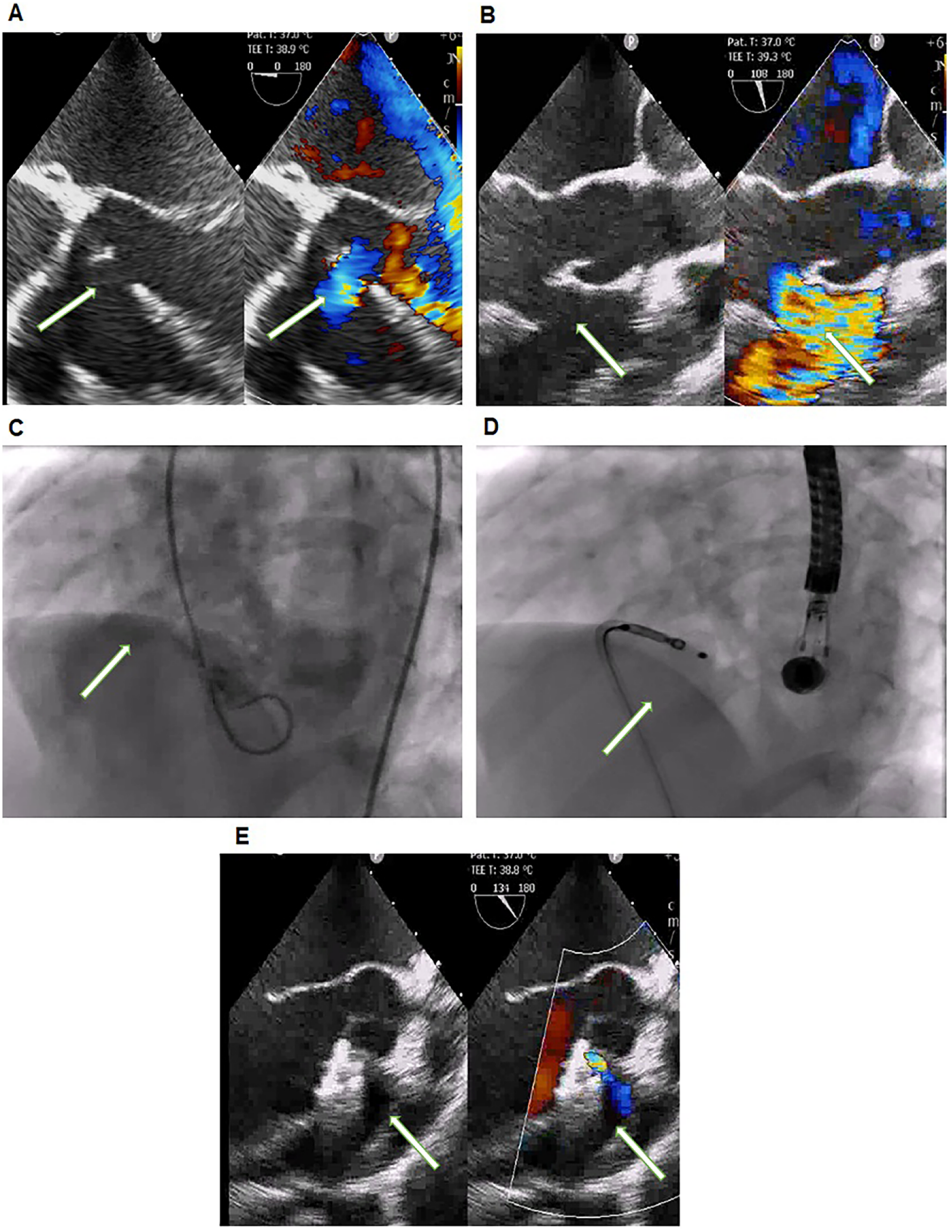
(A) TTE 0 degrees view with Doppler color and (B) TEE 110 degrees with Doppler color showing the perimembranous VSD (arrow). (C) Left Ventricular Angiography: the arrow shows the VSD. (D) The antegrade approach shows the device occluding the defect with the delivery cable attached to the RV disc. Post-device closure in (E) TEE 135 degrees with Doppler color showing a stable device with no significant residual flow (arrow).
